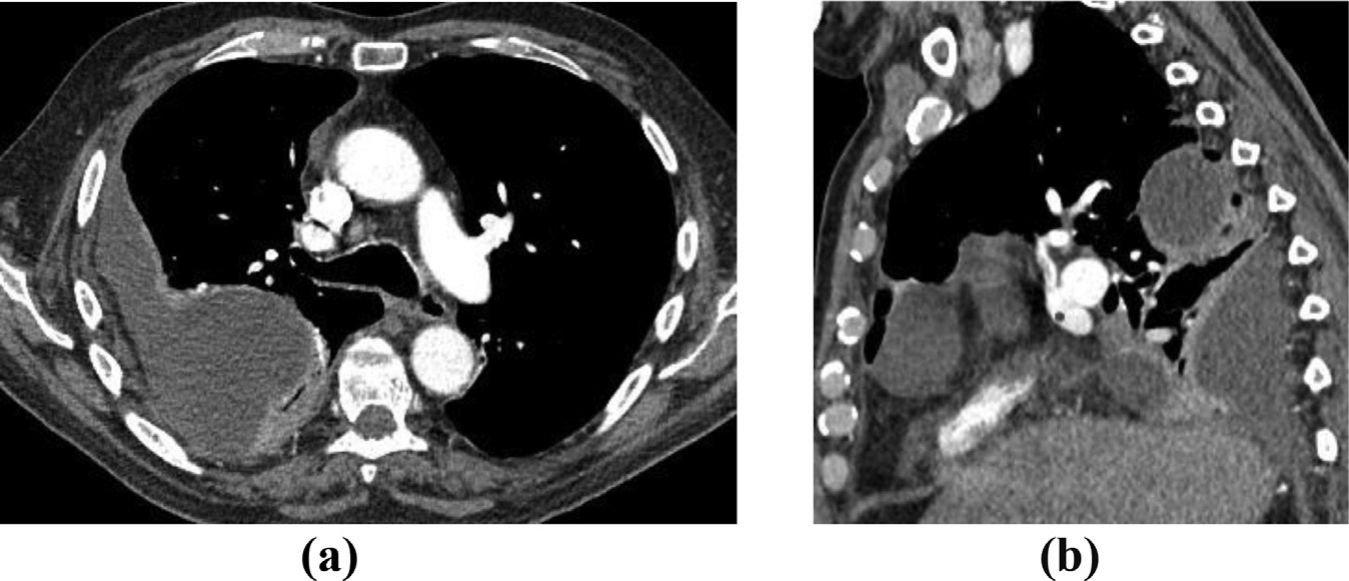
CT Thorax images in axial (a) and sagittal (b) planes showing a loculated right pleural effusion with enhancement of both layers of pleura suggesting an empyema.
